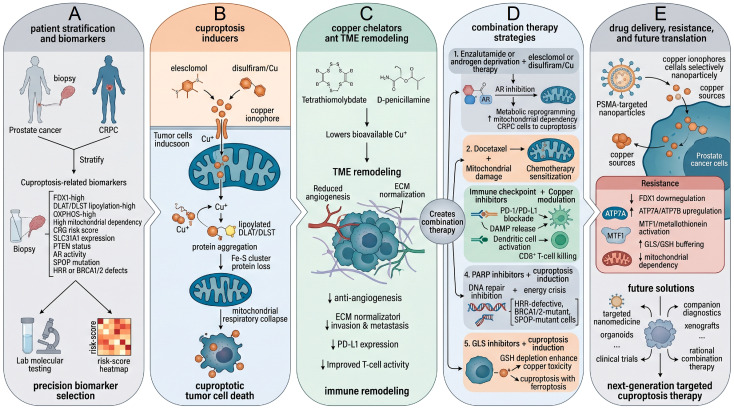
Therapeutic strategies targeting cuproptosis in prostate cancer. (A) Precision biomarker selection stratifies prostate cancer patients based on cuproptosis-related signatures (e.g., FDX1 status, DLAT/DLST lipoylation, and mitochondrial dependency) to identify optimal candidates for therapy. (B) Cuproptosis inducers, such as copper ionophores (elesclomol, disulfiram/Cu), shuttle copper into the mitochondria to trigger lipoylated protein aggregation, Fe-S cluster loss, and cuproptotic cell death. (C) Conversely, copper chelators induce systemic copper starvation, remodeling the tumor microenvironment through anti-angiogenesis, ECM normalization, and enhanced immune cell infiltration. (D) Rational combination strategies integrate cuproptosis modulation with standard-of-care therapies—including androgen receptor (AR) inhibition, chemotherapy, immune checkpoint inhibitors, PARP inhibitors, and GLS inhibitors—to induce synthetic lethality and overcome therapeutic resistance. (E) Future translational approaches highlight next-generation PSMA-targeted nanomedicine for specific drug delivery, alongside strategies to counteract acquired resistance mechanisms such as FDX1 downregulation or metallothionein activation.
